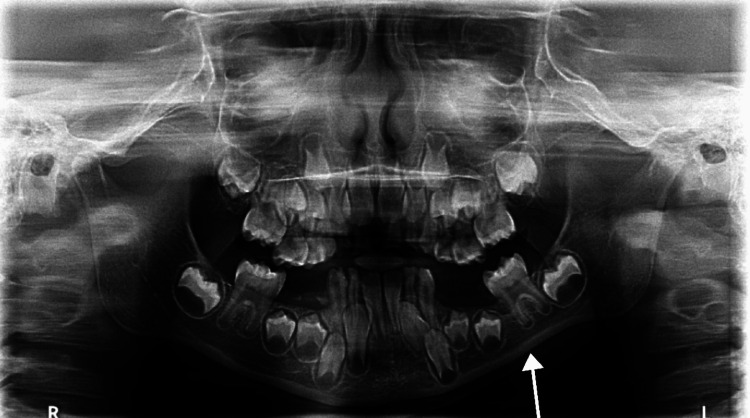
A panoramic X-ray showing a decreased alveolar bone height on the left side of the mandible
